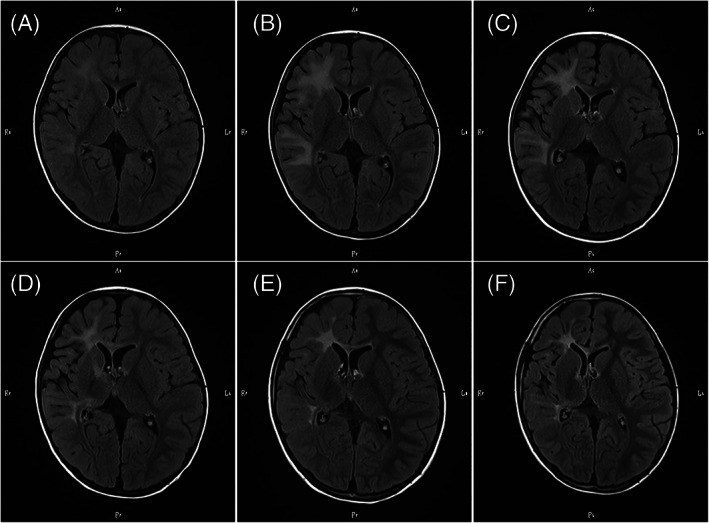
Change in axial fluid‐attenuated inversion recovery images on brain magnetic resonance imaging (MRI). (A) MRI scan at 1 year and 7 months of age, 2 months before unrelated cord blood transplantation (UCBT). Mild high‐signal intensity can be noted in the white matter of the right frontal and temporal areas. (B) MRI scan at 1 year and 9 months of age, 3 days before UCBT. The image shows expanded hyperintense lesions. (C) MRI scan at 1 year and 11 months of age, 2 months after UCBT. The scan revealed mild atrophy of the right frontal and temporal areas. (D) MRI scan at 2 years and 5 months of age, 8 months after UCBT. The image shows a slight decrease in right periventricular white matter. (E) and (F) MRI scan at 4 years of age, 3 years after UCBT and at 8 years of age, 7 years after UCBT. No progression was noted
